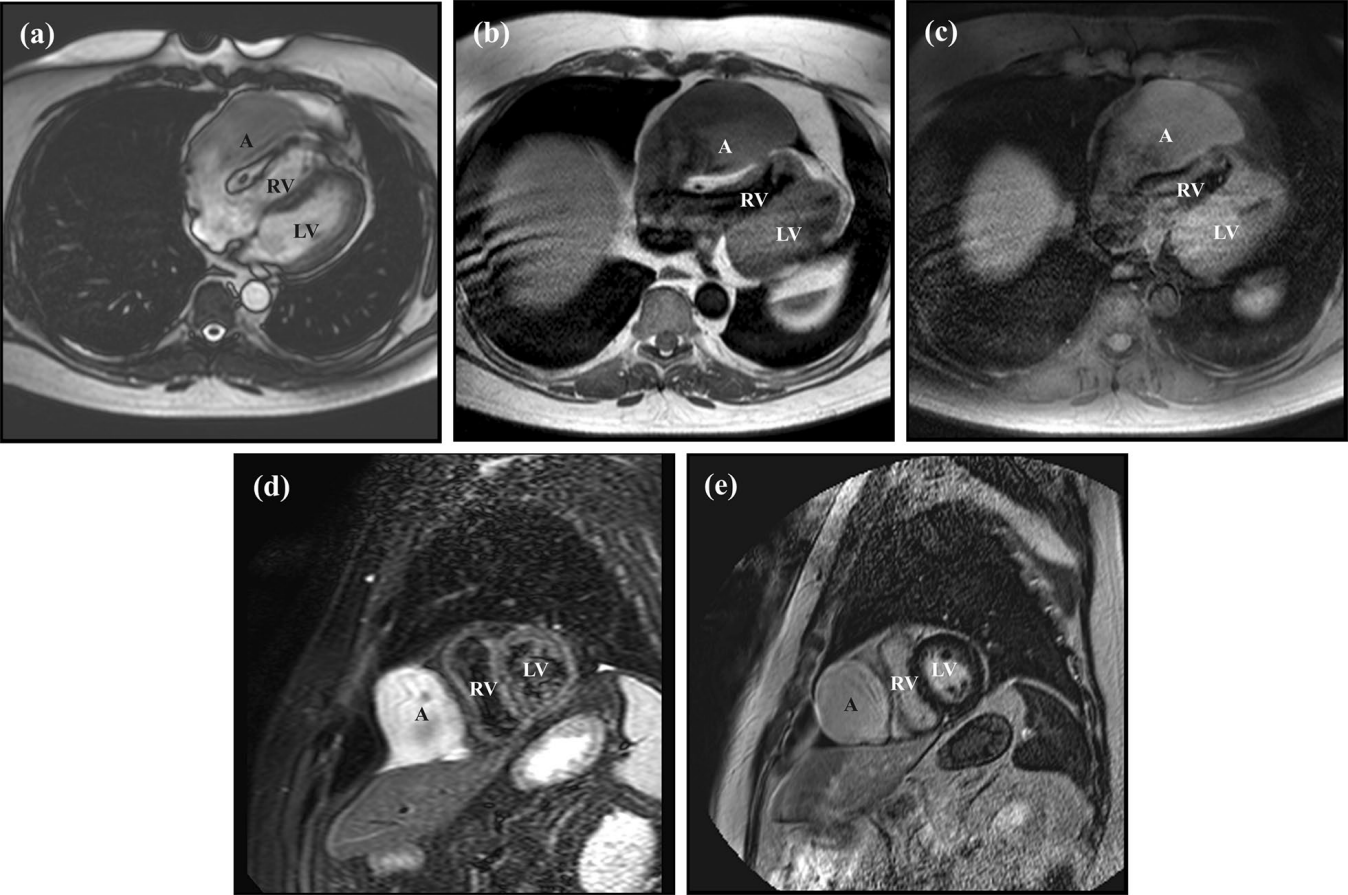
Additional cardiovascular magnetic resonance images centered at the right atrial appendage aneurysm. On balanced fast field echo using SENSE technique in axial orientation (a), the intraluminal signal of the RAA aneurysm is lower than that of the bright blood pool in the right and left ventricles, except for the area of high signal intensity at the right atrial appendage tip. On T1 spin echo in axial orientation without fat saturation (b) and with fat saturation (c) the blood in the right atrial appendage has intermediate signal intensity and does not follow the dark blood signal in the right ventricle. On the T2 short-tau inversion relaxation sax image (d), there is high signal intensity in the right atrial appendage in contrast to the blood pool in the ventricles on the post-contrast sax image (e), the signal in the RAA is similar to the blood pool in the right ventricle and left ventricle. There is no wall or intraluminal enhancing component. A, RAA aneurysm; RV, right ventricle; LV, left ventricle
